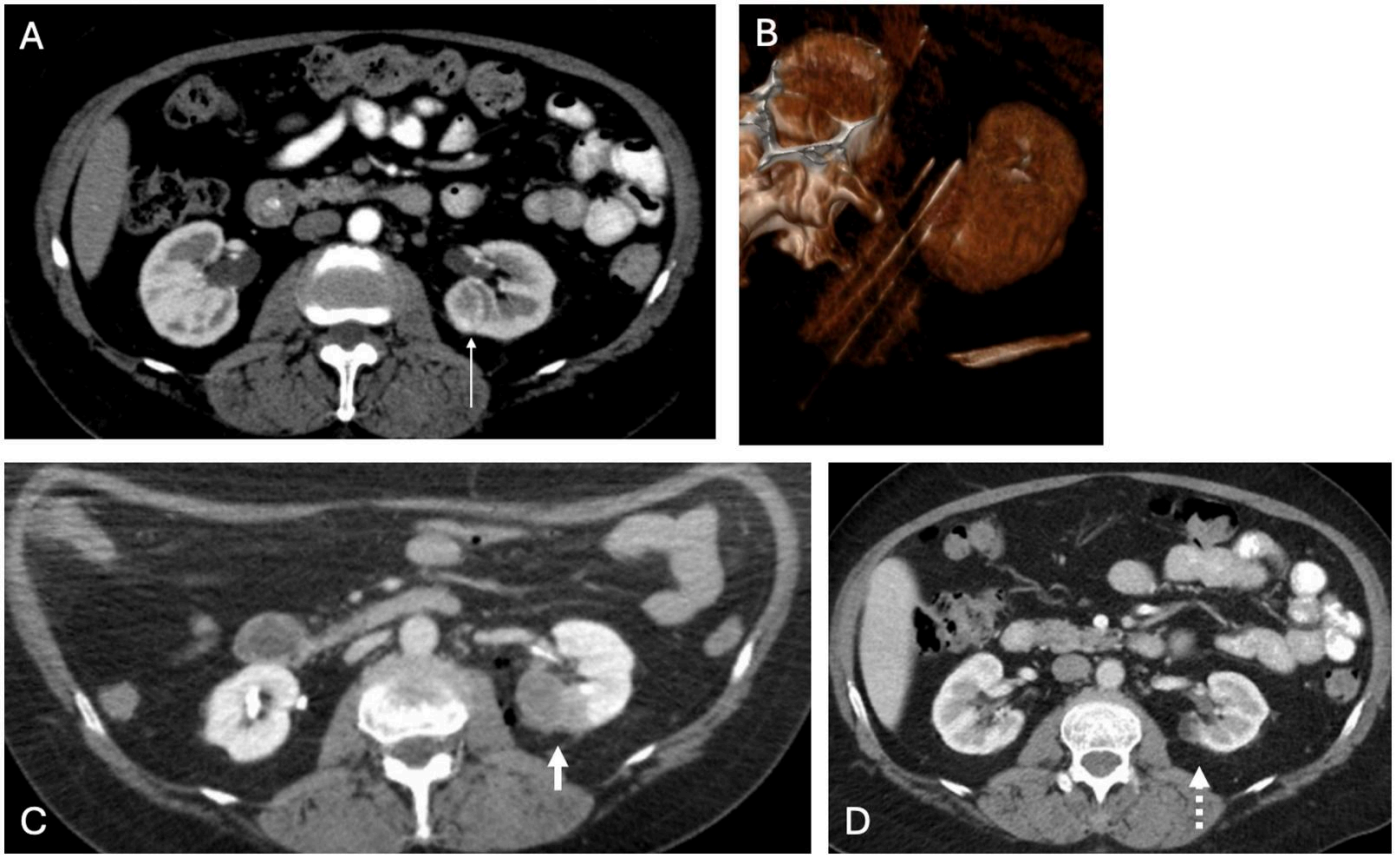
Irreversible electroporation (IRE) in renal cell carcinoma. IRE was performed in a 74-year-old female with left renal cell carcinoma: (A) axial contrast-enhanced CT in the early arterial phase demonstrates a 2.7 cm enhancing mass (white arrow) in the left kidney; (B) maximum intensity projection and 3D reconstructed image demonstrate 4 needles placed in parallel for IRE; (C) tumor enhancement is absent immediately following IRE (thick white arrow); (D) at 4-year follow-up, the tumor remains non-enhancing (dotted white arrow) with no residual tumor or recurrence.
